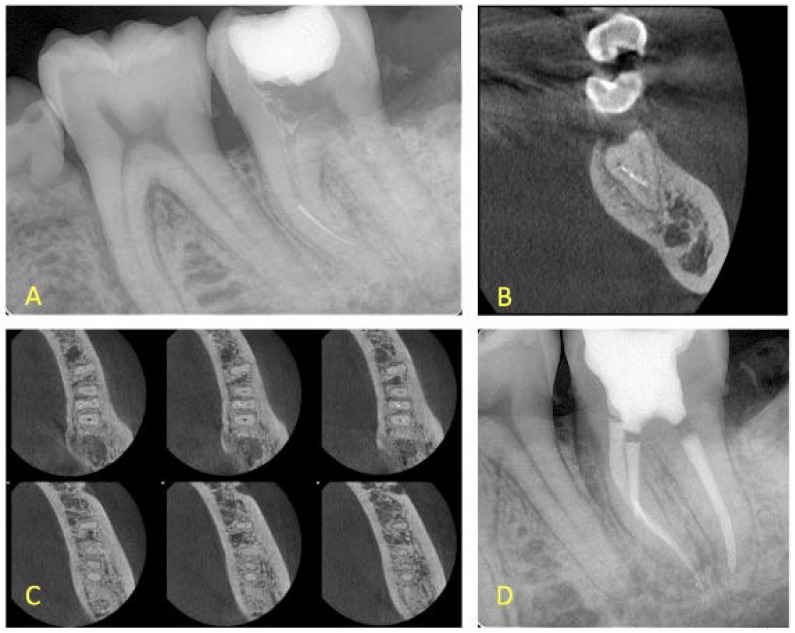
(A) Initial periapical radiograph of a mandibular second molar with an instrument fracture at the middle-apical third of the mesial root, (B,C) Coronal and axial views demonstrating that the fragment is located at the mesiolingual canal and the two canals are merging at the apical third, (D) Final radiograph in which the fragment has been removed with no excess dentin removal.
